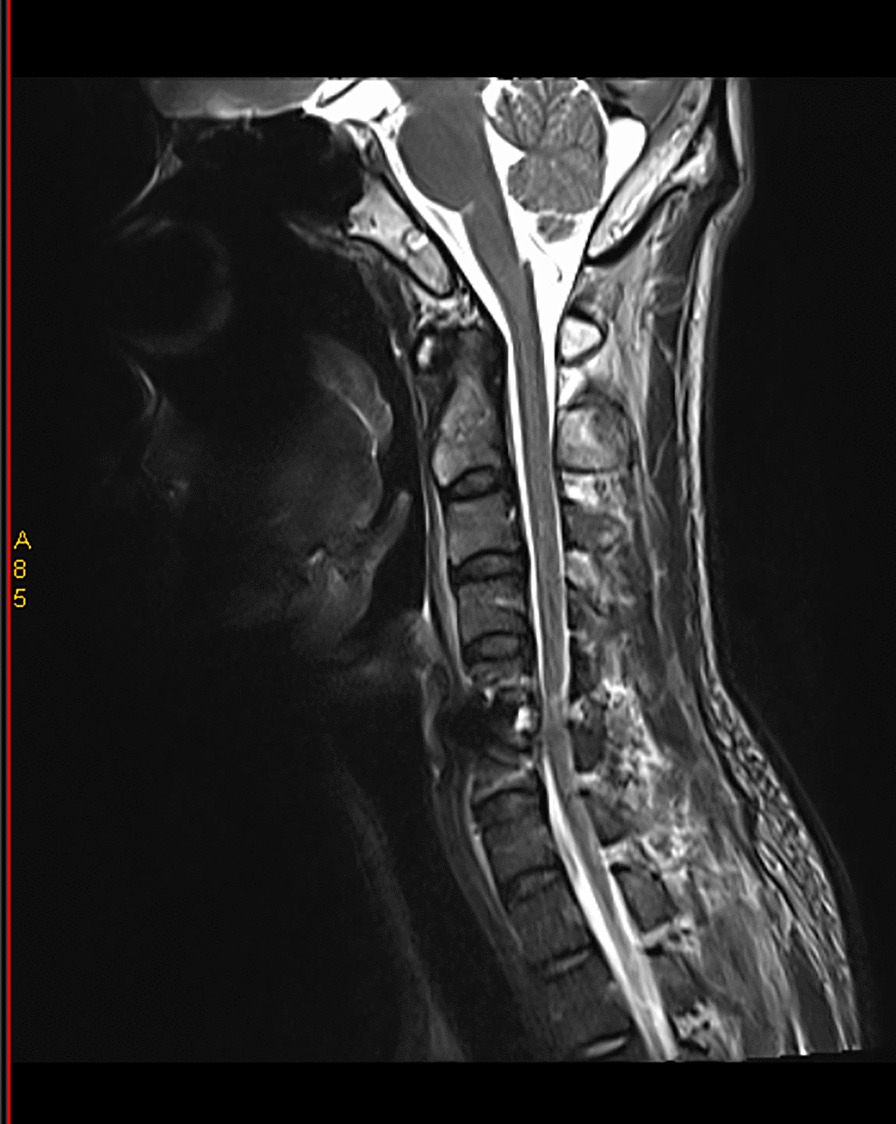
Postoperative MRI showed that giant cervical disc herniation had been removed
